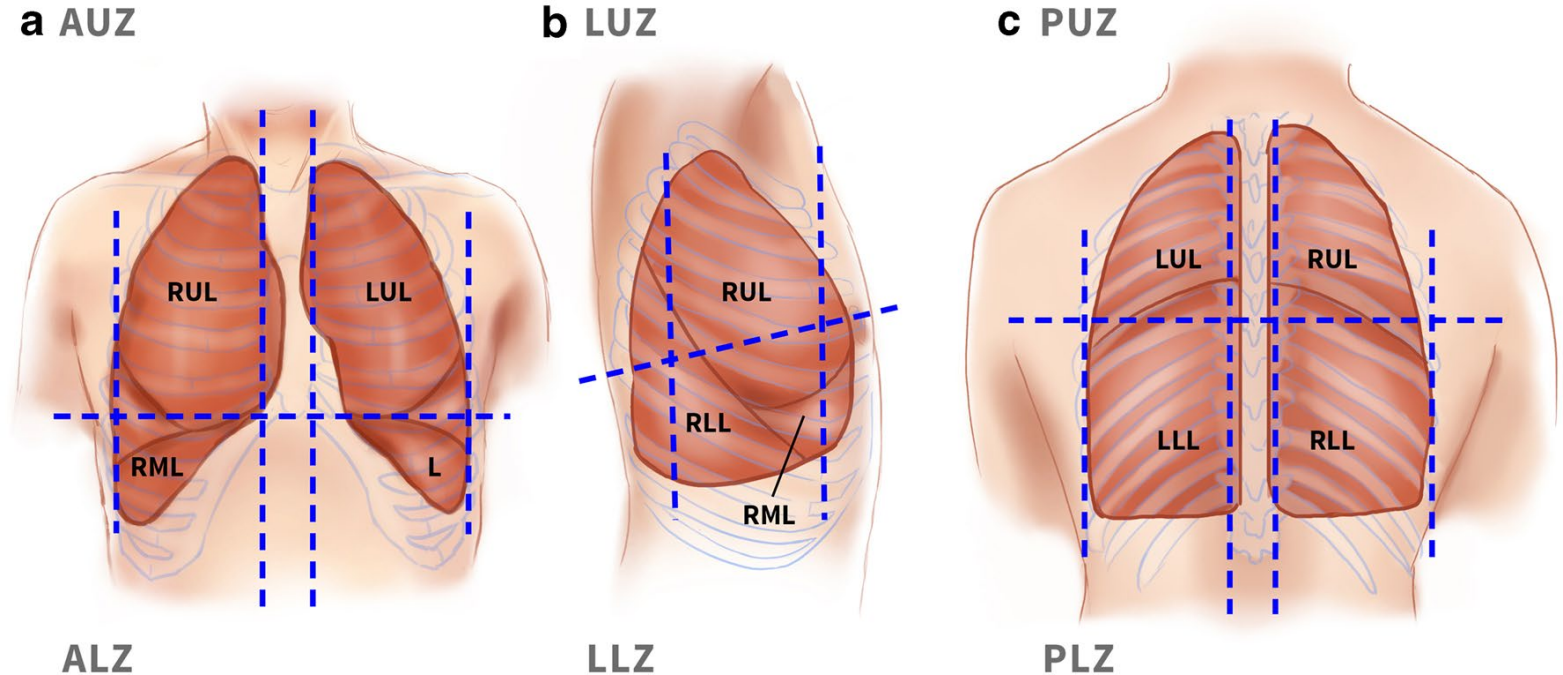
Surface anatomy of the regions of the lung. Ultrasound surface anatomy: the parasternal, anterior axillary and posterior axillary lines divide the chest wall into anterior, lateral and posterior regions. a The anterior region, delineated superiorly by the clavicle, inferiorly by the liver or the spleen, medially by parasternal line and laterally by the anterior axillary line. The anterior region is further divided by an arbitrary line to anterior upper zone and anterior lower zone. b The lateral region, delineated superiorly by the axilla, inferiorly by the liver or the spleen, anteriorly by the anterior axillary line and posteriorly by the posterior axillary line. An arbitrary line further divides the lateral region to lateral upper zone and lateral lower zone. c The posterior region, delineated by the posterior axillary line laterally and paravertebral line medially. A horizontal arbitrary line at the tip of the scapula further divides the posterior region to upper and lower zone. *ALZ* anterior lower zone, *AUZ* anterior upper zone, *L* lingula, *LLL* left lower lobe, *LLZ* left lower zone, *LUL* left upper lobe, *PLZ* posterior lower zone, *PUZ* posterior upper zone, *RLL* right lower lobe, *RML* right middle lobe, *RUL* right upper lobe
